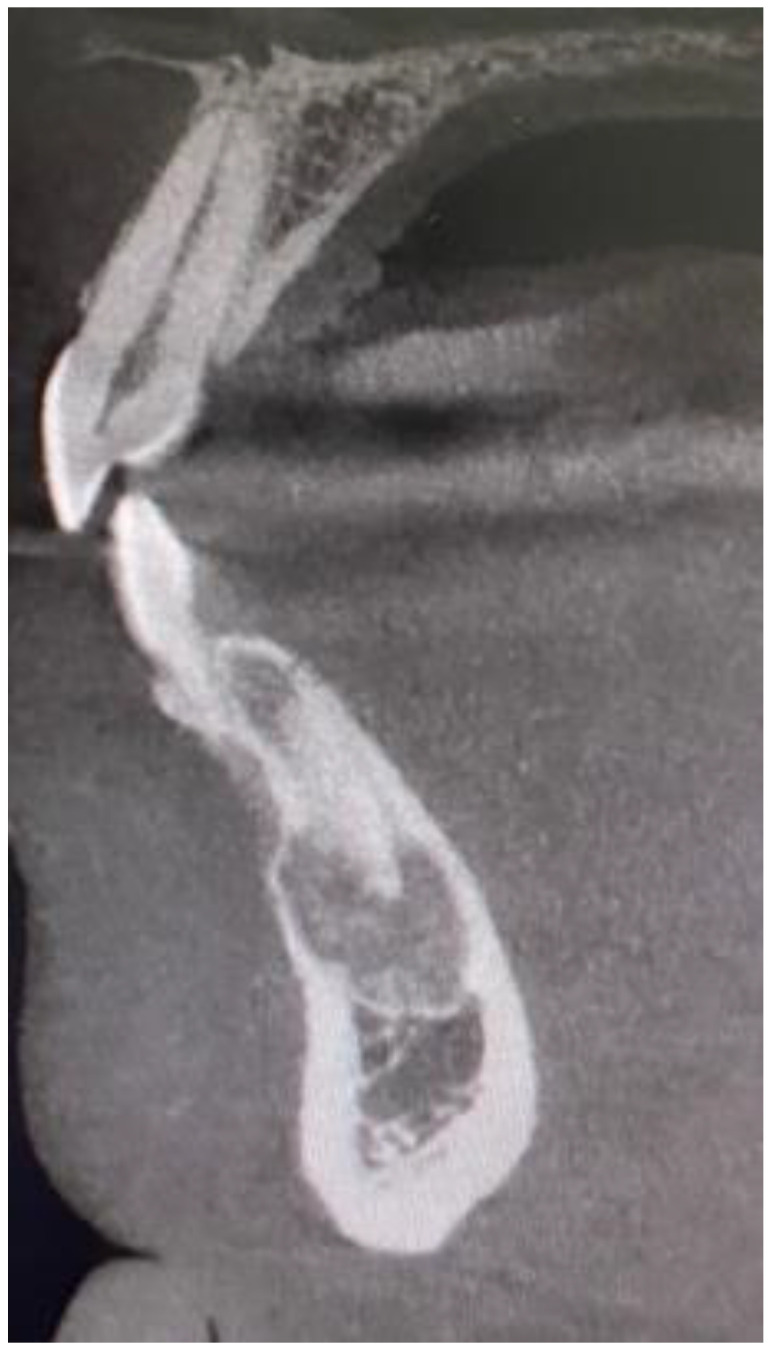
CBCT sagittal view of COD at the mandibular anterior symphysis region.
